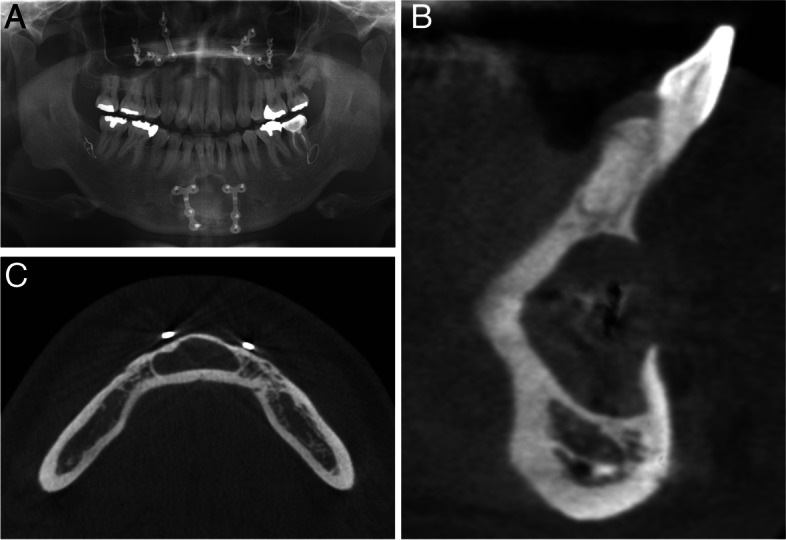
Preoperative radiographs. A Panoramic view. B Sagittal cone-beam computed tomographic (CBCT) image. C Axial CBCT image. A radiolucent lesion in the anterior mandible with two plates and labial cortical bone perforation were observed
